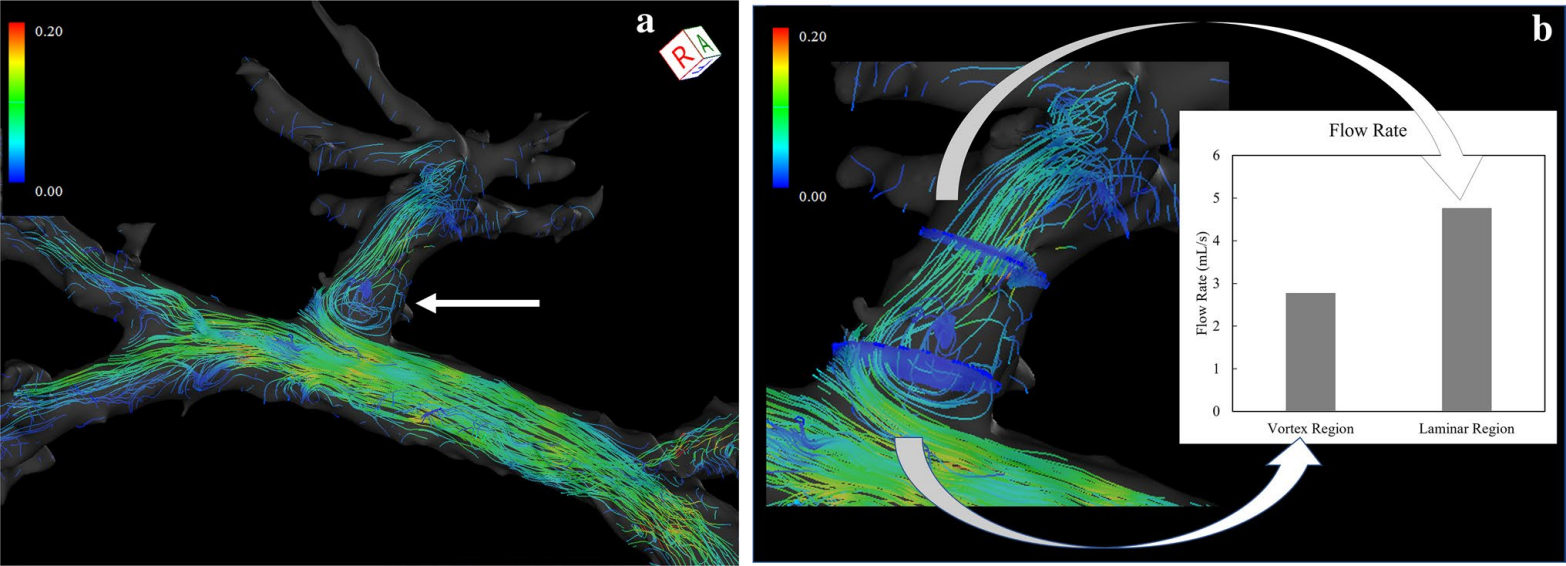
a Vortex flow. A vortical streamline is seen at the root of the left portal vein, a finding of vortex flow (arrow). b “Retrospective flowmetry” of the vortex flow at the left portal venous root and laminar flow distal to the left portal vein. Blood flow is underestimated in areas of vortex flow than in areas of laminar flow. Doppler ultrasonography and 2D cine phase-contrast MRI cannot retrospectively avoid vortex areas, which is an advantage of 4D Flow MRI
